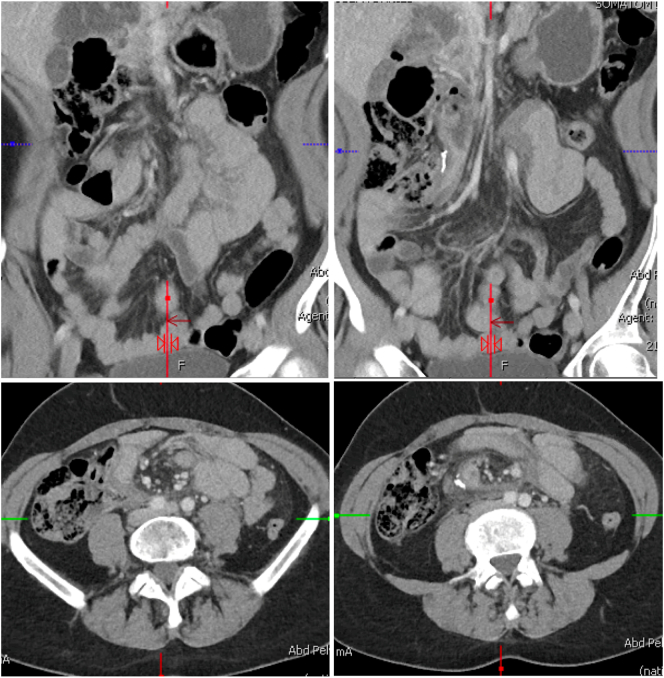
CT images from ED on hospital admission day 1 showing mesenteric swirling, SBO, and possible midgut volvulus.
